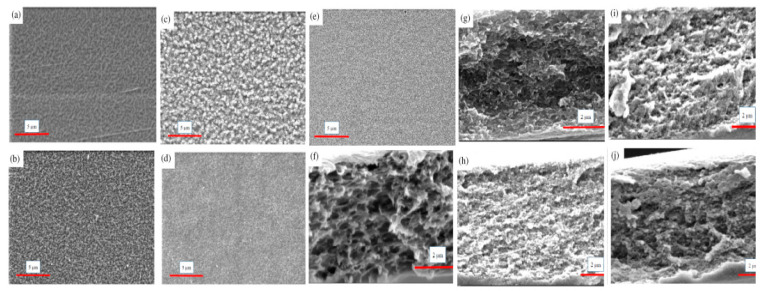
SEM surface images of (a) CTA pristine, (b) CTA-1 wt.% SiO_2_, (c) CTA-2 wt.% SiO_2_, (d) CTA-3 wt.% SiO_2_, (e) CTA4 wt.% SiO_2_ membranes, SEM cross-sectional images of (f) CTA pristine, (g) CTA-1 wt.% SiO_2_, (h) CTA-2 wt.% SiO_2_, (i) CTA-3 wt.% SiO_2_, and (j) CTA-4 wt.% SiO_2_ of membranes. Reprinted with permission from Ref. [286]. Copyright 2020 Prihatiningtyas I. et al.
